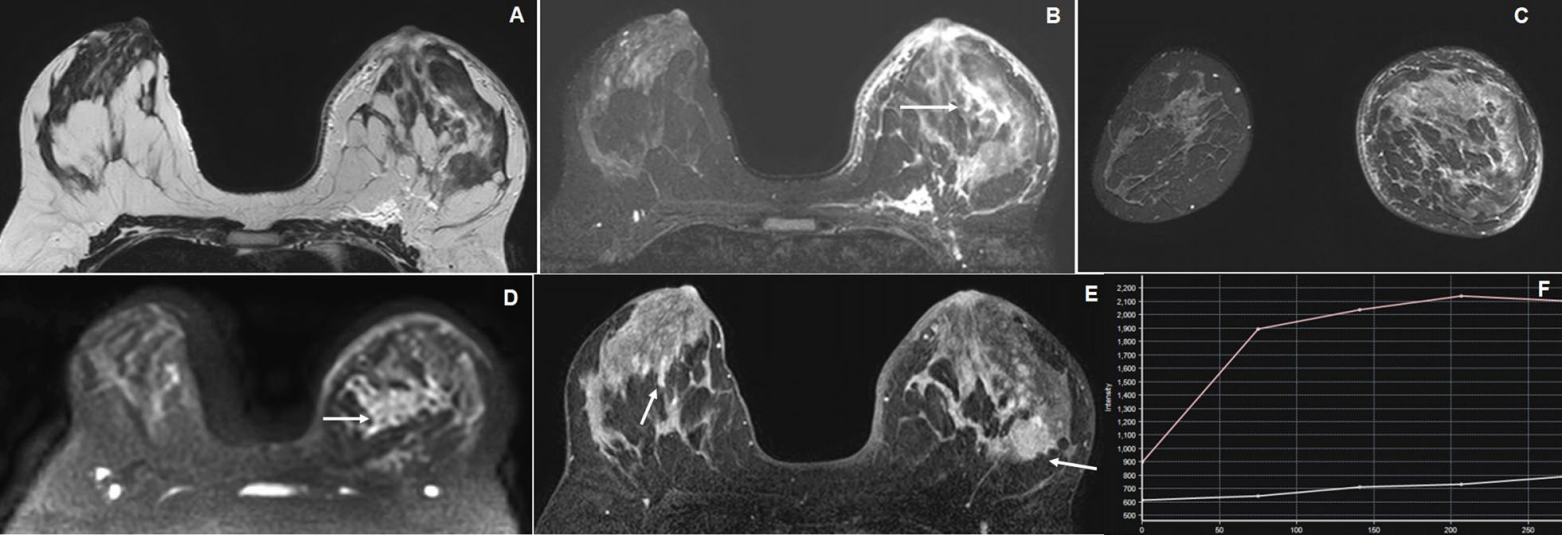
A 45-year-old female presented with a left breast lump. A, B and C Axial T2W, STIR and coronal STIR images reveal increased signal intensities in fibroglandular tissues of left breast, dominantly in superior and inferolateral quadrants (arrow) causing enlargement of left breast with thickening of overlying skin. D Axial DWI image shows a heterogeneous diffuse pattern of hyperintensities in the left breast (arrow) with fine nodular hyperintensities in the right breast. E and F Axial dynamic post-contrast (DCE-MRI) image reveals irregular nodular enhancement of the thickened fibroglandular tissues in both breasts, more on the left side (arrows) where the kinetic curve in the nodular enhanced area shows slow progressive enhancement curve (type I). These lesions were labeled as BI-RADS 2 lesion and were found to be chronic granulomatous mastitis
